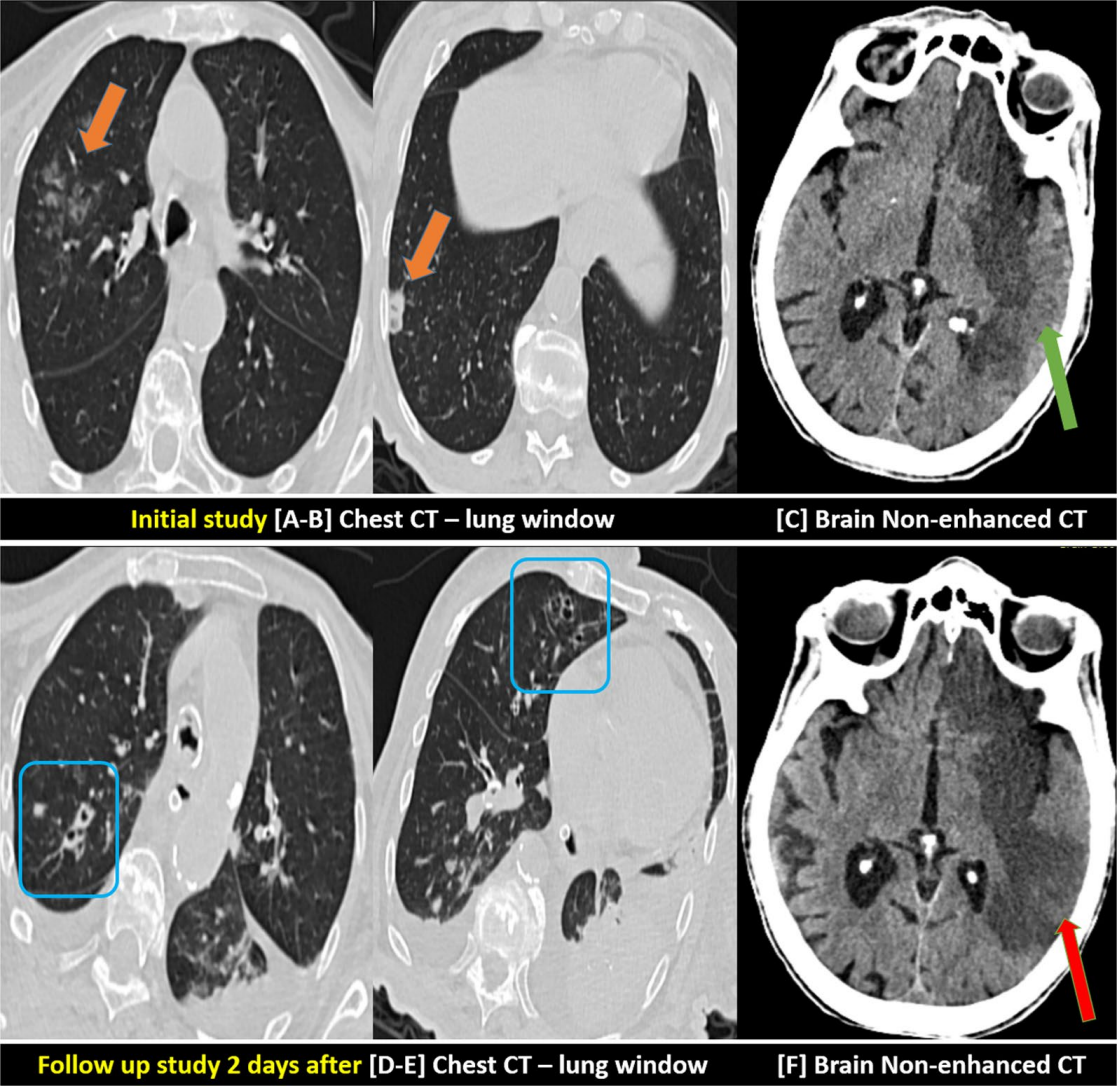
An 85-years-old female patient with COVID-19 infection complicated by invasive aspergillosis and cerebrovascular stroke: A–C Initial study: A, B Axial chest CT images (lung window) showing confluent right upper lobar ground-glass patches and right basal sub-pleural small cavitating consolidation (orange arrows). C Axial brain CT image showing large left fronto-tempro-pareital recent hypo-dense infarction along with the territory of the left ACA and MCA (partially sparing left temporal cortical area … green arrow). D–F Follow-up study after 2 days: D, E Axial chest CT images (lung window) showing denovo bilateral pleural collection and cavitating lung nodules (blue squares). F Axial brain CT image showing size progression of the previous large infarction involving more volume of the left temporal lobe (red arrow)
